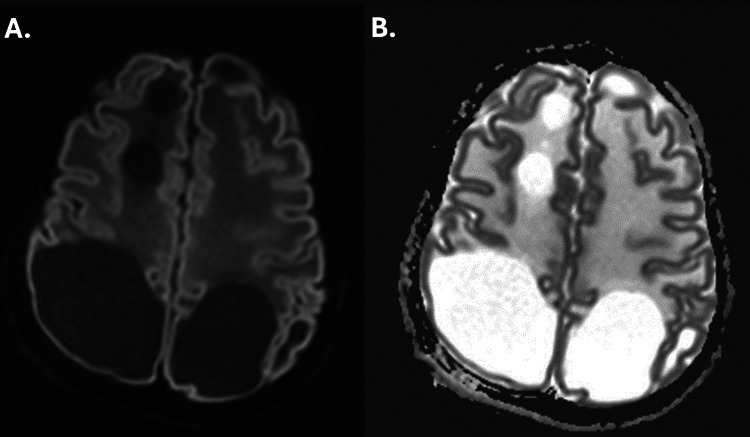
(A) Axial DWI 3-T and (B) axial ADC 3-T MRI images showing subcortical cysts in the bilateral occipitoparietal regions. No evidence of diffusion restriction is seen in the deep white matter anterior to the above-mentioned cysts, consistent with vasogenic edema. DWI, diffusion-weighted imaging; ADC, apparent diffusion coefficient
